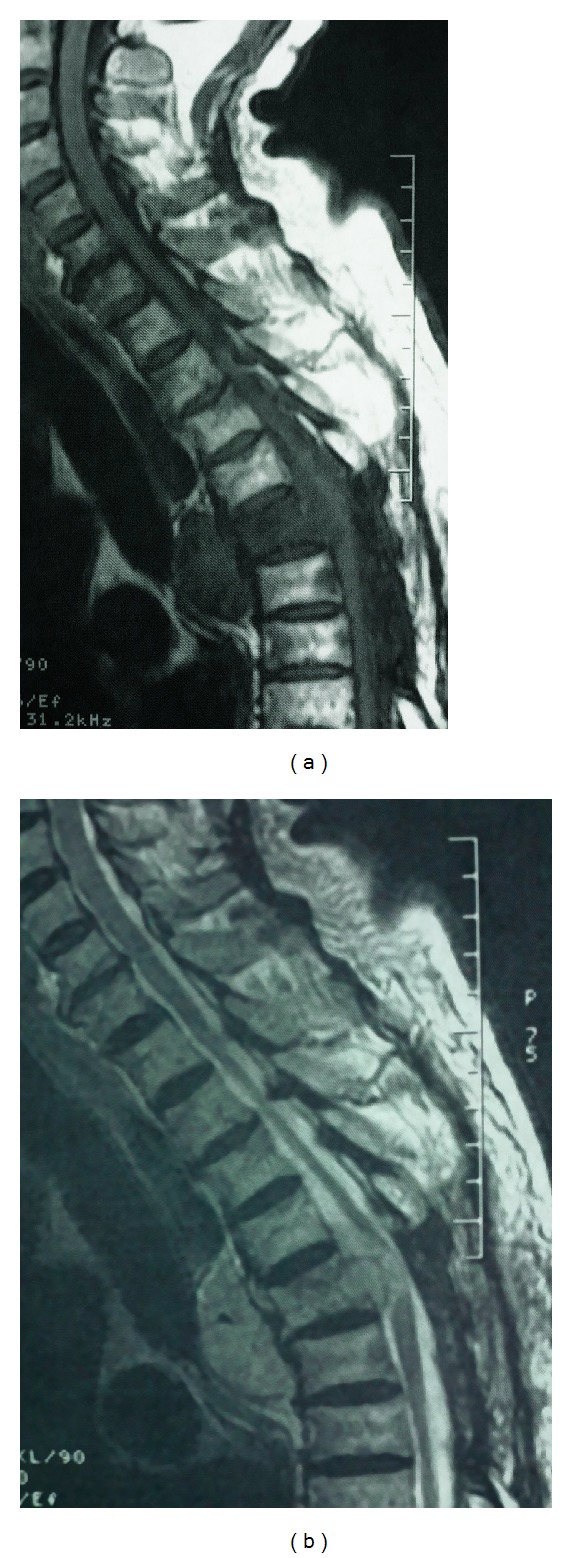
Recurrence sagittal T1-weighted (a) and T2-weighted (b) MR images showing an important space occupying mass with spinal cord compression, T5 vertebral body involvement, and large posterior mediastinum extension.
